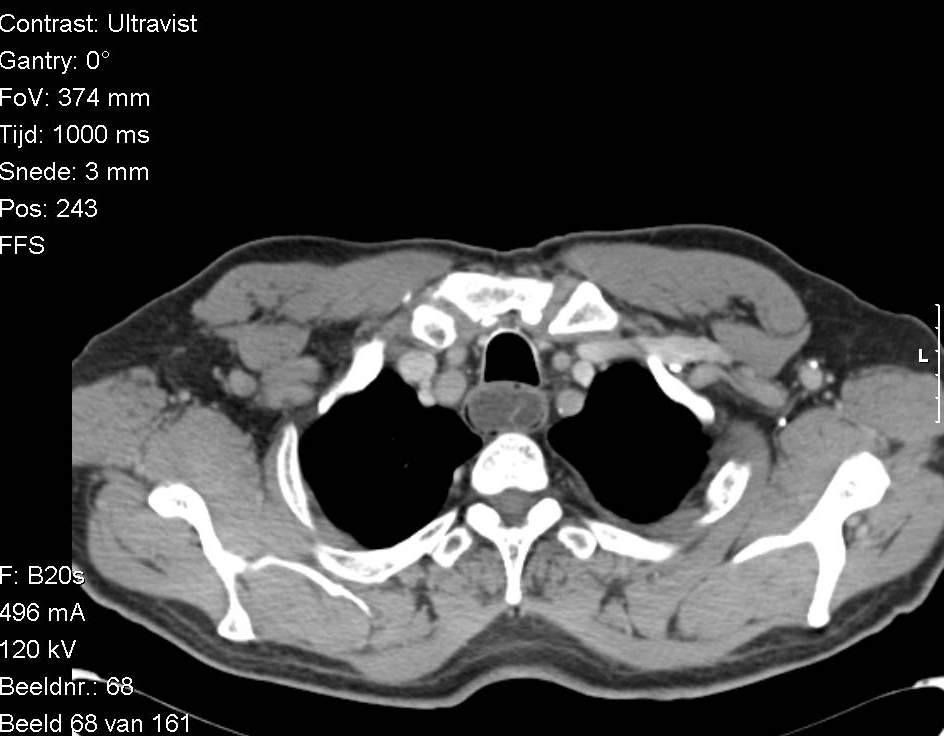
CT scan showing the large polyp in the esophagus nearly occluding the lumen.
